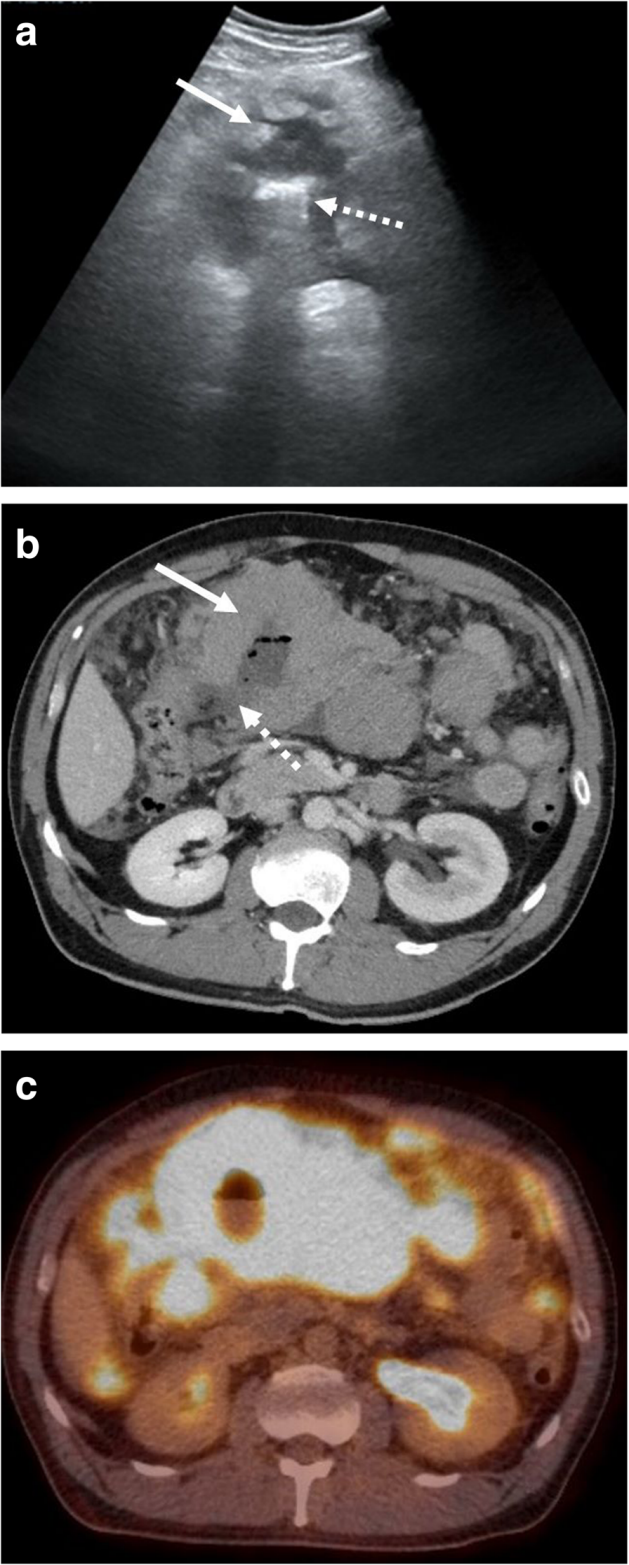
Bowel involvement with perforation. A 49-year-old male presenting to the emergency department with abdominal pain. a Transverse grayscale ultrasound image demonstrates mass-like thickening of a bowel loop within the mid-abdomen (solid arrow) with central air and associated dirty shadowing (dashed arrow). b Subsequently performed axial CT shows a large central mass (solid arrow) with associated perforation (dashed arrow). c Follow-up fused axial PET-CT demonstrates associated hypermetabolic activity and extensive background abdominal disease. Burkitt lymphoma was confirmed at surgery
